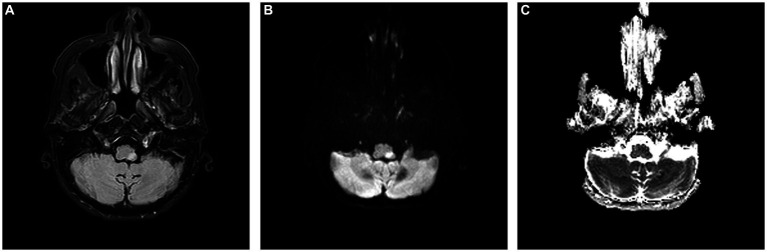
Brain MRI. Acute ischemia of the left medulla oblongata. (A) FLAIR transverse image, (B) DWI transverse image, (C) ADC transverse image.
